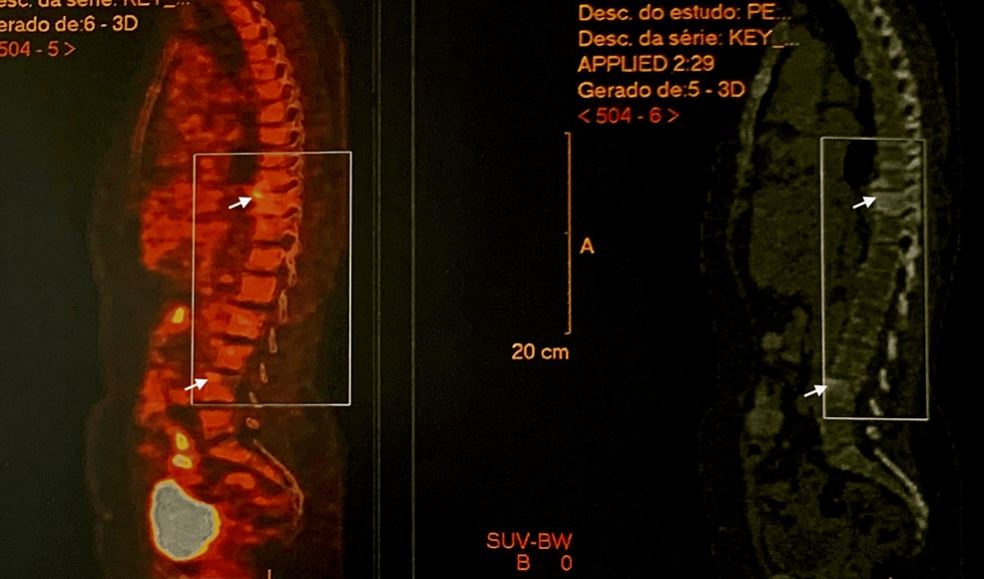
PET/CT scan showing multiple skeletal lesions affecting the spine with low to moderate 18F-FDG uptake (white arrows).
